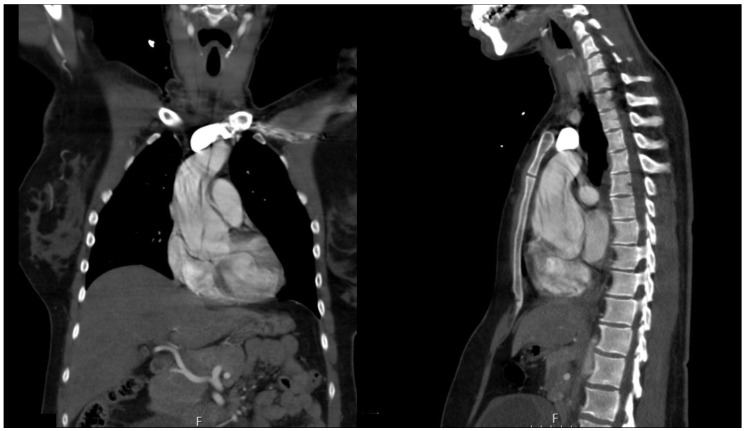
CT imaging with contrast agent of a pregnant woman, 27th gestational week.
